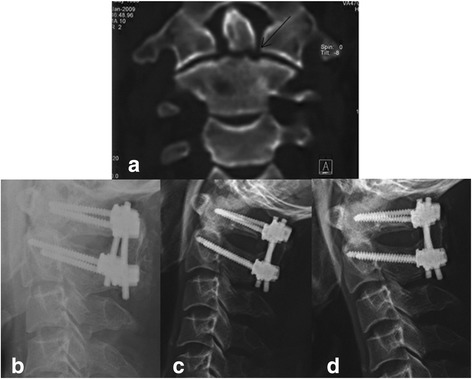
A 46-year-old man had type II odontoid fracture caused by traffic accident. a Preoperative computed tomography (CT) showed fracture line was located at the bottom of odontoid (*arrow*). b Lateral radiograph obtained on the 1st day after the surgery showed the position of C1 lateral mass–C2 pedicle screws and the autogenous bone block. c, d Dynamic lateral radiographs showed bone fusion in the 3rd month postoperatively
